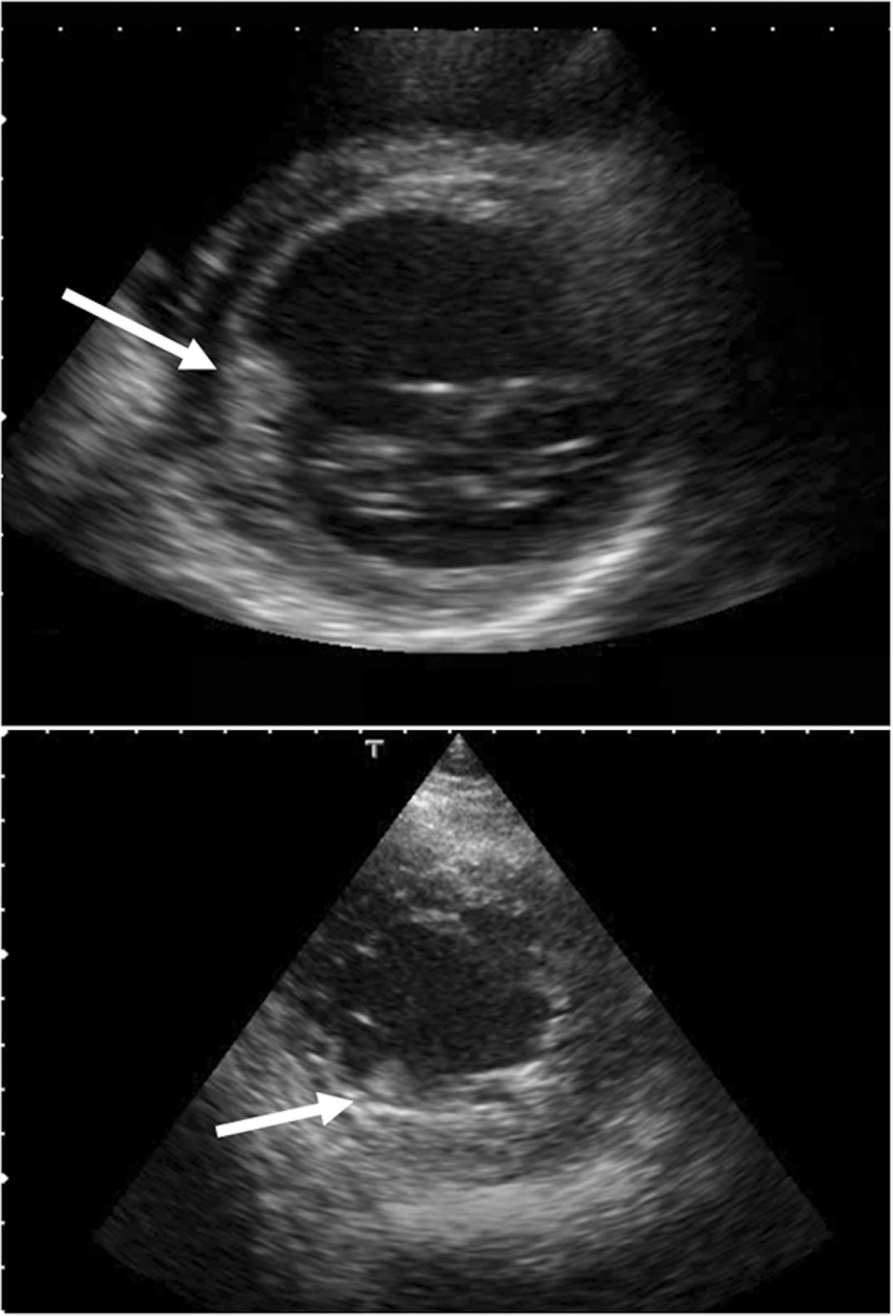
Short-axis view echocardiograms. Arrows point to two mobile thrombi.
